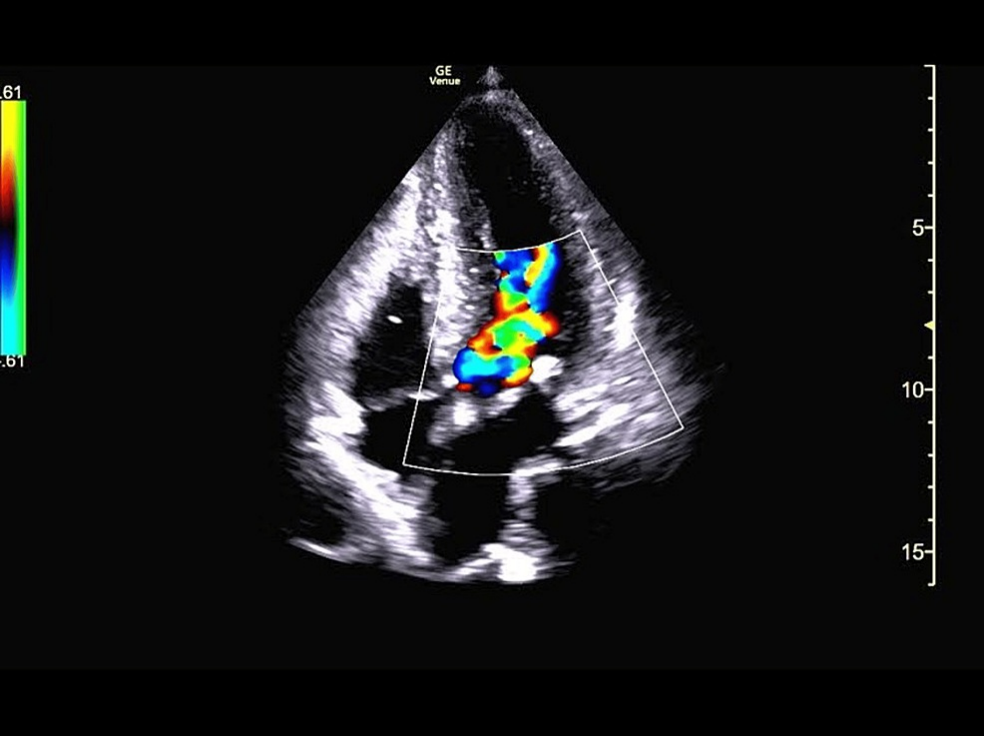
Apical four-chamber view revealing turbulence (aliasing with mixed color Doppler signal) within the LV outflow tract LV: Left ventricle.
